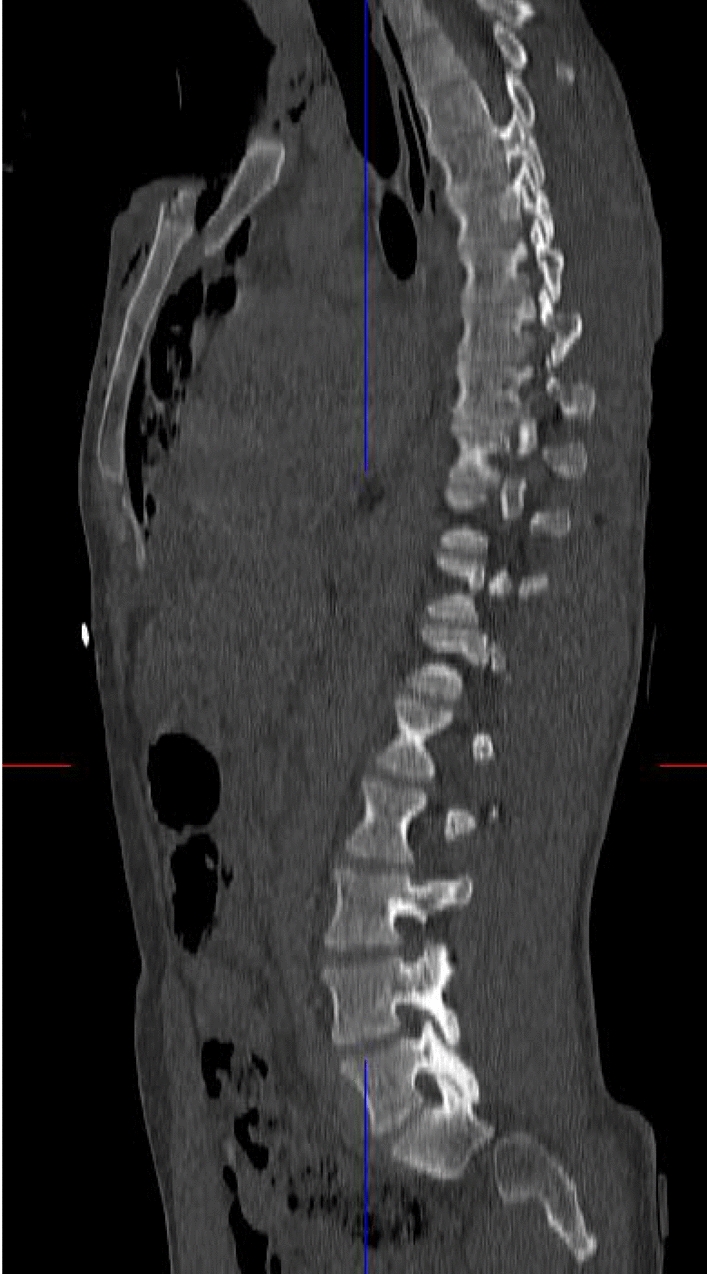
Sagittal plane of the CT showing the proximal fragment of the manubrium sterni being dislocated posteriorly. No injuries of the spine were detected
